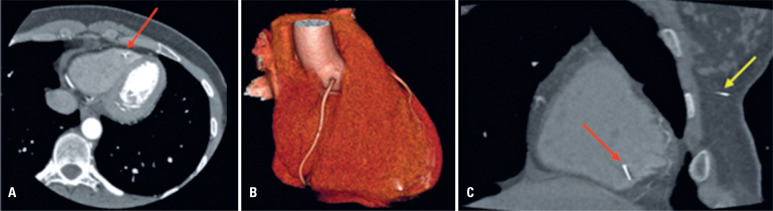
A) Computed tomography angiography (CTA) shows a metallic material in the apical region of the right ventricle (red arrow); B) CTA three-dimensional reformatting displaying the metallic fragment in the same location; C) CTA oblique plane reformatting showing that the linear metallic image within the right ventricle (red arrow) is similar to the one in the subcutaneous tissue of the left breast (yellow arrow)
